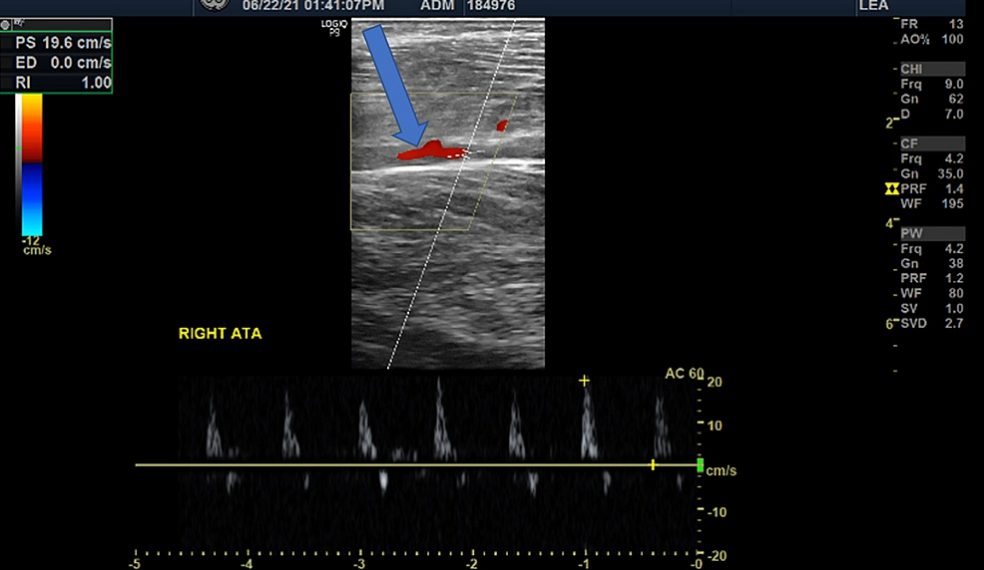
Doppler image of right anterior tibial artery shows presence of blood flow (blue arrow)
